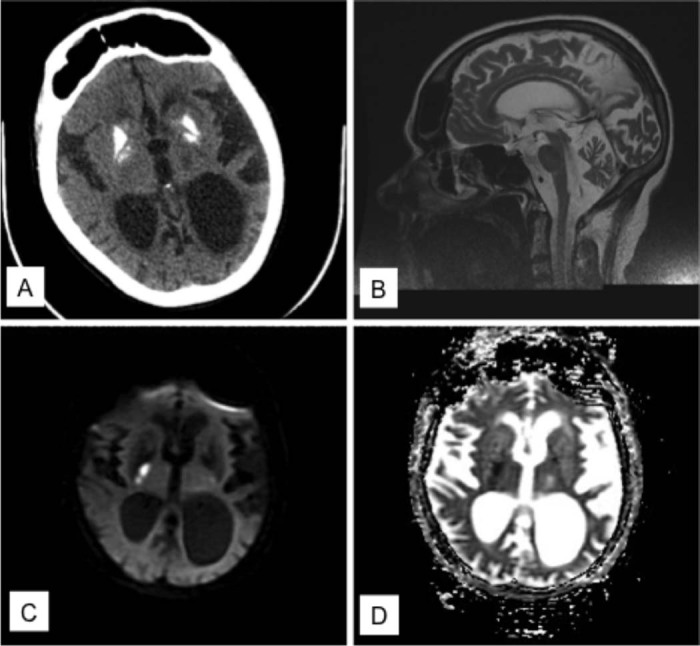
Neuroimaging results (A) CT head showed bilateral, symmetrical basal ganglia calcification. (B) Sagittal view of cranial MRI showed significant cerebral and cerebellar atrophy at the age of 39 years. (C) Diffusion-weighted imaging showed restricted diffusion in the right internal capsule and lentiform nucleus with corresponding low signal change in (D) ADC map at the age of 44 years.
